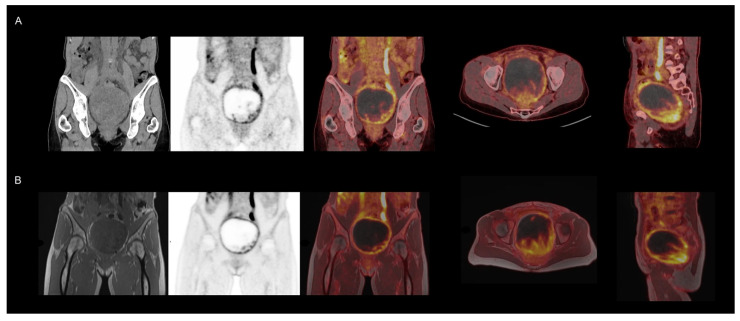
Regional focused imaging—female pelvis. Suspected recurrent disease in a patient with prior surgery for carcinosarcoma in the right ovary/adnexa. PET/CT (A) and BIOGRAPH One PET/MRI (B) revealed a heterogeneously metabolically active tumor suggesting recurrence, subsequently confirmed by surgery. PET acquisition was performed using continuous bed motion at 1.5 mm/s, corresponding to approximately 3 min per field of view (FOV) for PET/CT, compared to 21 min for a single-bed PET/MRI acquisition.
